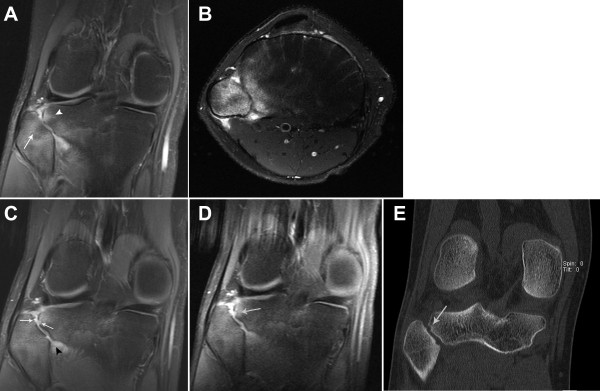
Progression of right proximal tibiofibular joint (TFJ) arthritis. (A) Coronal fat-saturated (FS) proton density MRI of the right knee shows bone marrow edema within the lateral aspect of the tibial plateau (arrowhead) and the fibular head (arrow). (B) Axial FS T2 sequence of the same joint demonstrating diffuse marrow edema of the fibular head and lateral tibial plateau. There is also adjacent soft tissue edema and a small amount of fluid within the proximal TFJ. (C) Follow-up coronal FS proton density sequence demonstrating an erosion at the site of prior bone marrow edema (arrows) as well as persistent inflammatory changes within the proximal TFJ (arrowhead). (D) Follow-up coronal FS T1 post gadolinium contrast-enhanced sequence again demonstrating the erosion seen in (C) (arrow) as well as enhancement of proximal TFJ synovium. (E) Reformatted coronal CT image of the same joint delineates the erosion at the lateral tibial plateau (arrow).
